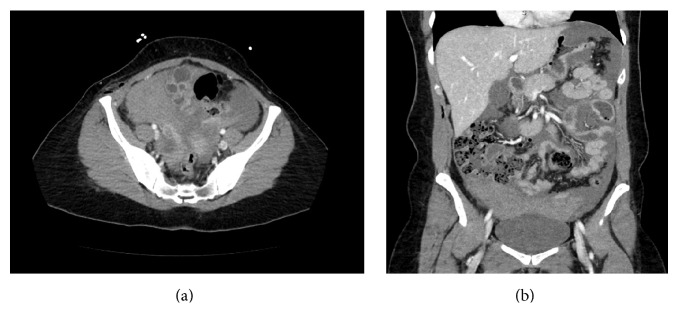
Axial (a) and coronal (b) abdominal CT scan showing hemoperitoneum.
